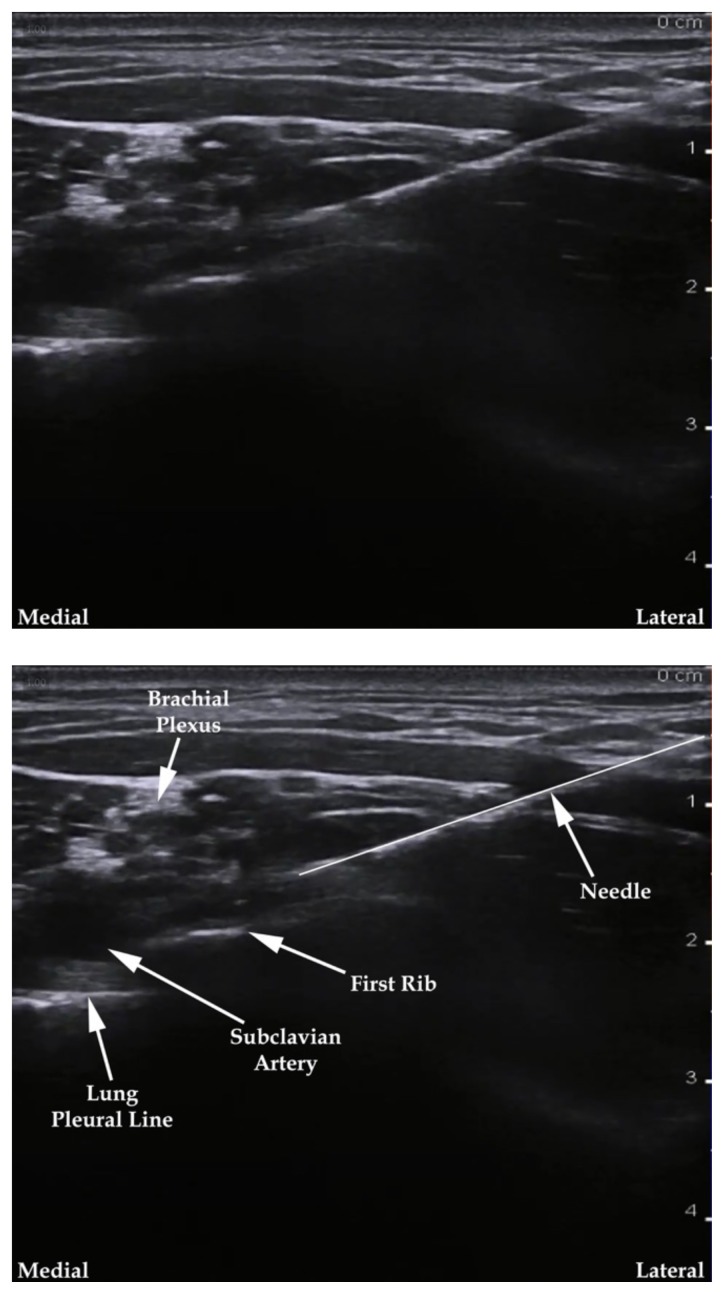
Ultrasound-guided supraclavicular brachial plexus nerve block. Modified from source.^13^
